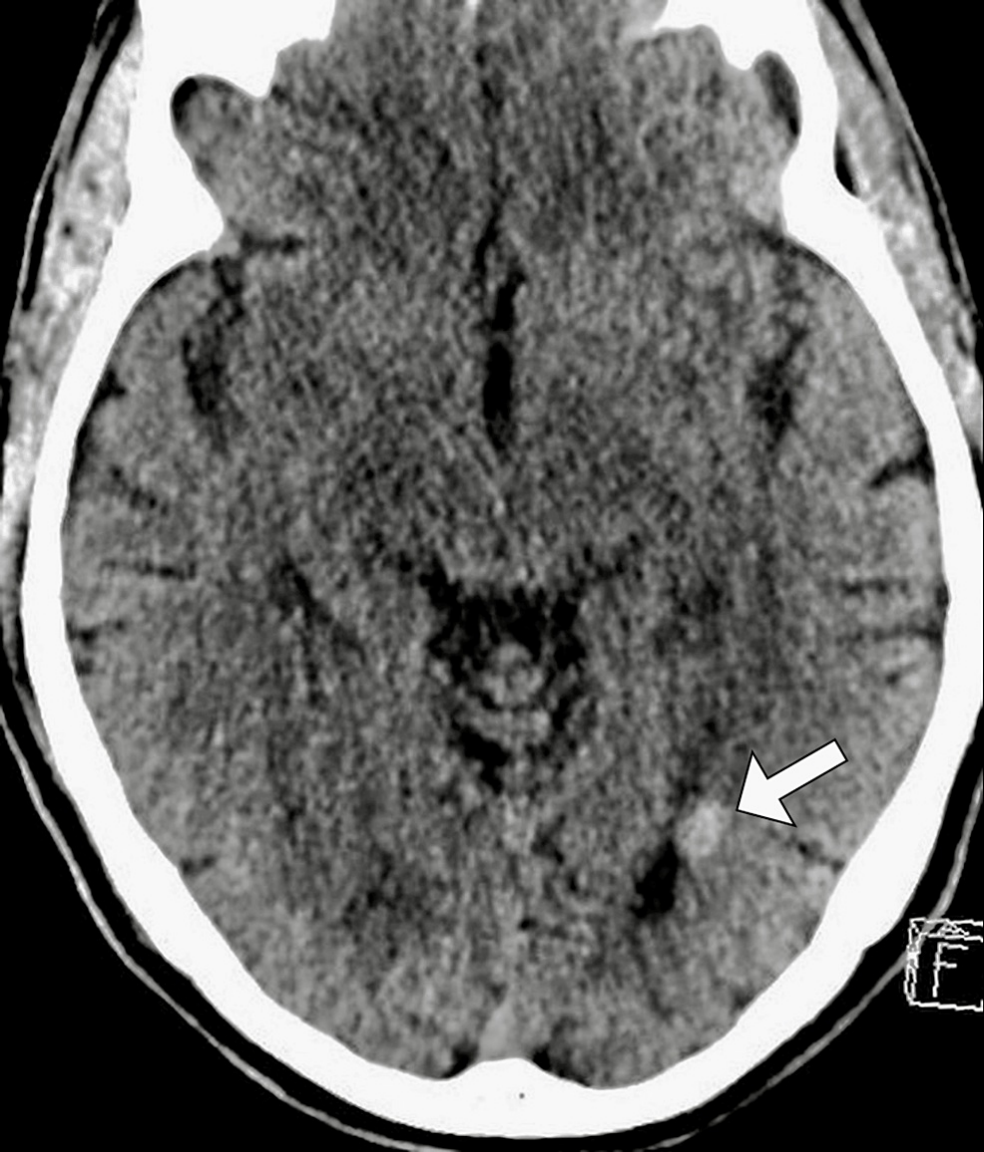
Axial CT of the head revealing a hyperdense nodule (arrow) adjacent to the occipital horn of the left lateral ventricle.
